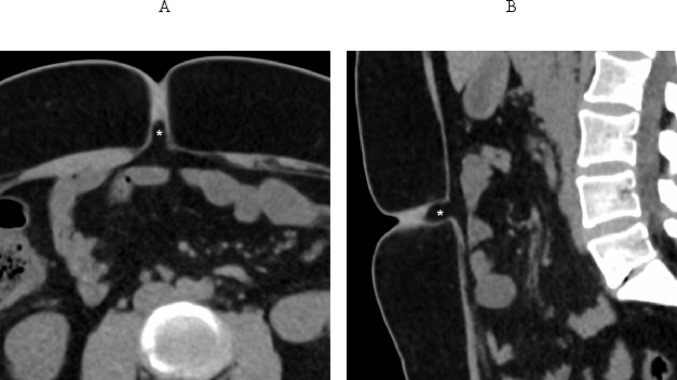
Axial (A) and sagittal (B) CT images demonstrate a fat-density tract (*) extending between the medial borders of the rectus abdominis muscles, posterior to the navel and anterior to the parietal peritoneum, without evidence of a peritoneal-lined sac or visceral herniation, increasingly labeled as an “umbilical hernia” in current radiology reports, however better described as the the umbilical canal
